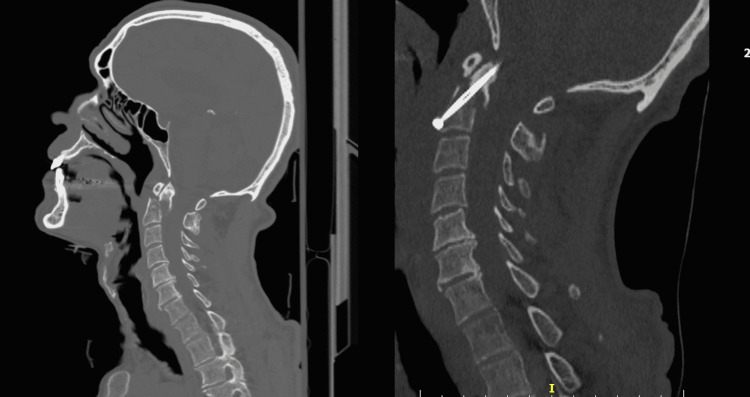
Pre and postoperative computed tomography images of the cervical spine. Left image: posteriorly dislocated type II odontoid fracture with significant step off and evident bony spike. Right image: postoperative single screw fixation with satisfactory reduction.
